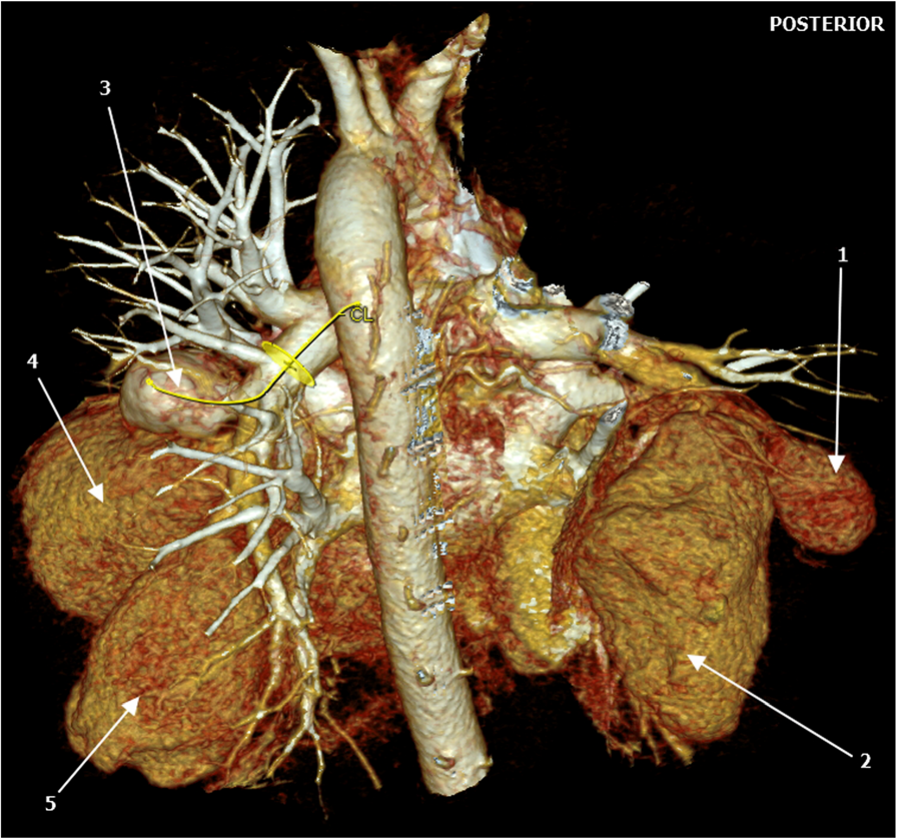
Volume-rendered computed tomography image showing multiple bilateral pulmonary pseudoaneurysms (*arrows*)
